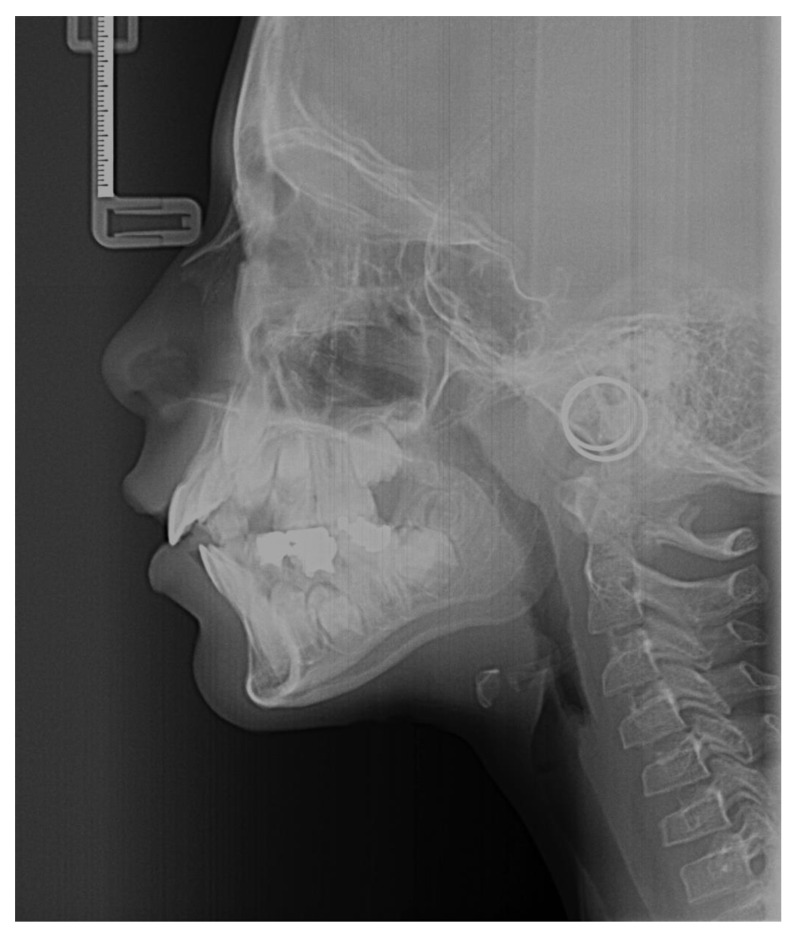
A sample of lateral cephalometric radiographs.
